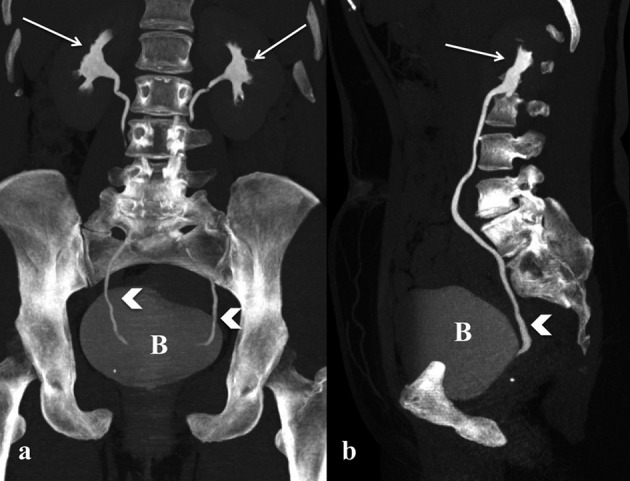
a-b: MIP reconstruction obtained in coronal and sagittal plane. CT Urography shows the iodinate urine in the renal pelvis (white arrow), ureter (white arrowhead) and bladder (B).
